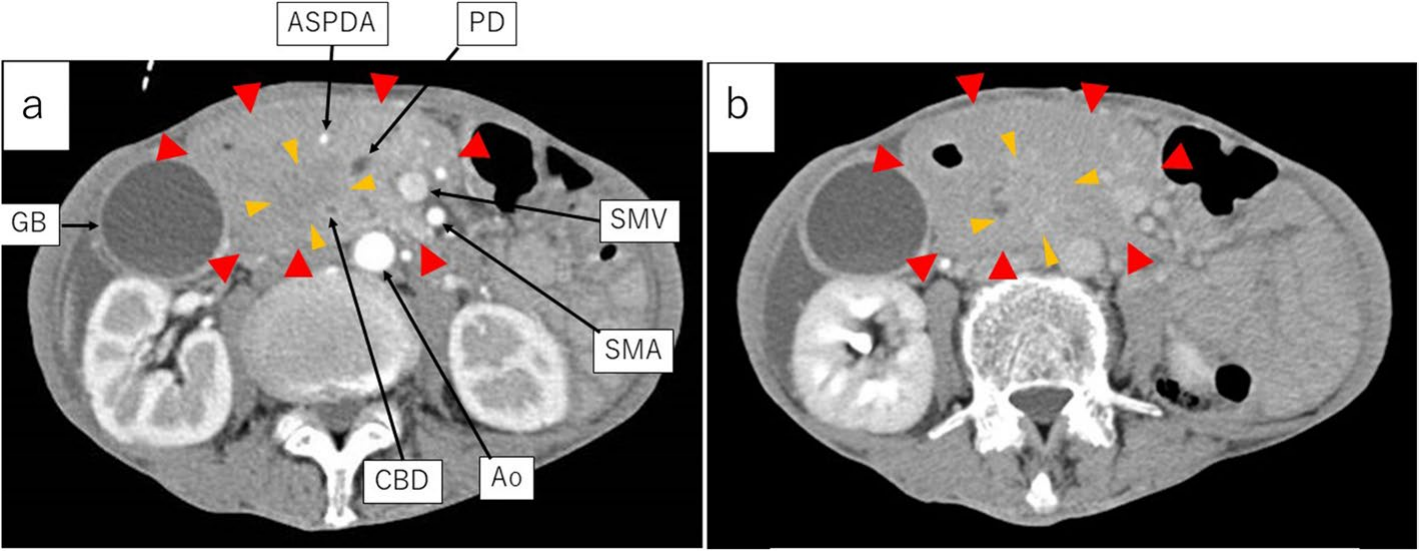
Contrast-enhanced CT (axial view); a Early phase. b Late phase. Contrast-enhanced CT revealed that the peripheral part had a slightly low density in the early phase and homogeneous isodensity in the late phase. However, the central part revealed a lower density in the early phase and a heterogeneous high density in the late phase. Ao aorta, ASPDA anterosuperior pancreaticoduodenal artery, CBD common bile duct, Du duodenum, GB gall bladder, SMA superior mesenteric artery, SMV superior mesenteric vein, PD pancreatic duct, PV portal vein
